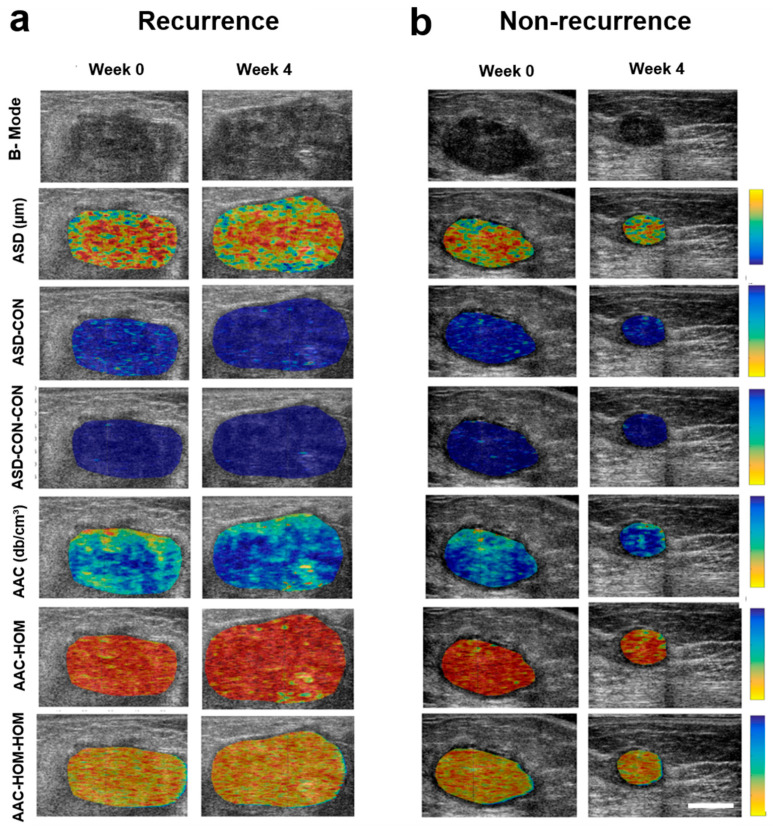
Quantitative ultrasound parametric images for representative patients with and without disease recurrence. Representative ultrasound B-mode images and QUS-derived parametric maps (ASD, ASD-CON, ASD-CON-CON, AAC, AAC-HOM, AAC-HOM-HOM) from one patient each with recurrence (a) and no recurrence (b) acquired at week 0 and week 4 of treatment. The color maps represent the quantitative values of the spectral parameters within the tumor. The change in values of the parameters with treatment can be appreciated by the change of assigned color to the sub-regions of interest within the tumor. The color scale on the right side represents the range for individual features, ASD parameter of 40 to 200 µm, ASD-CON texture feature of 0 to 20, ASD-CON-CON texture derivative of 0 to 54, AAC parameter of 7 to 65 db/cm^3^, AAC-HOM texture feature of 0 to 1, AAC-HOM-HOM texture derivative of 0 to 1. The scale bar represents 2 cm.
